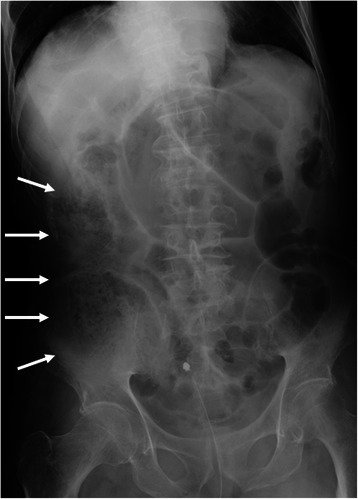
An abdominal X-ray. In the spine position, an irregular gas image was observed in the right lateral abdomen, corresponding to pneumoretroperitoneum (arrows)
